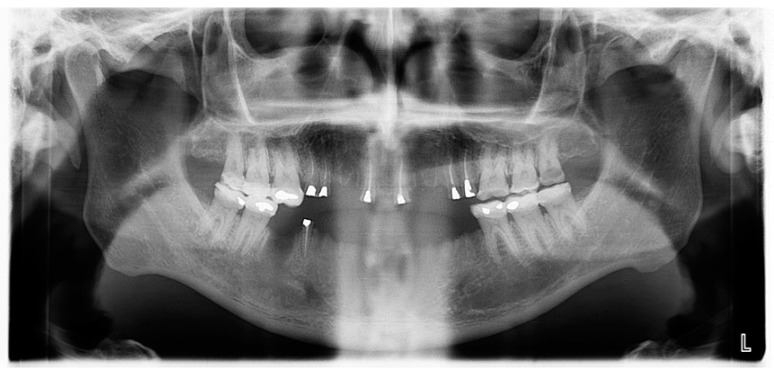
Panoramic radiograph after procedures performed under general anesthesia.
